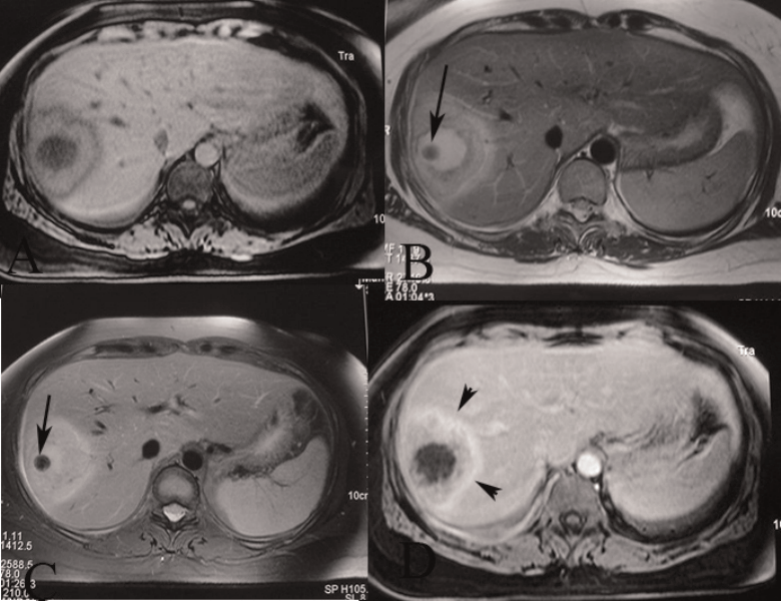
Axial MR images reveal (a) a central hypo-intense lesion on vibe, and hyperintensity on (b) T2-weighted and (c) STIR, with enchanced halo on gadolinium-enhanced images. (d) Periabscess liver tissue is hypointense on T1-weighted and mildly hyperintense on T2-weighted images, and reveals increased enhancement on post-gadolinium images (arrowheads). Note the dense calcium deposit as a low signal intensity lesion (arrows).
